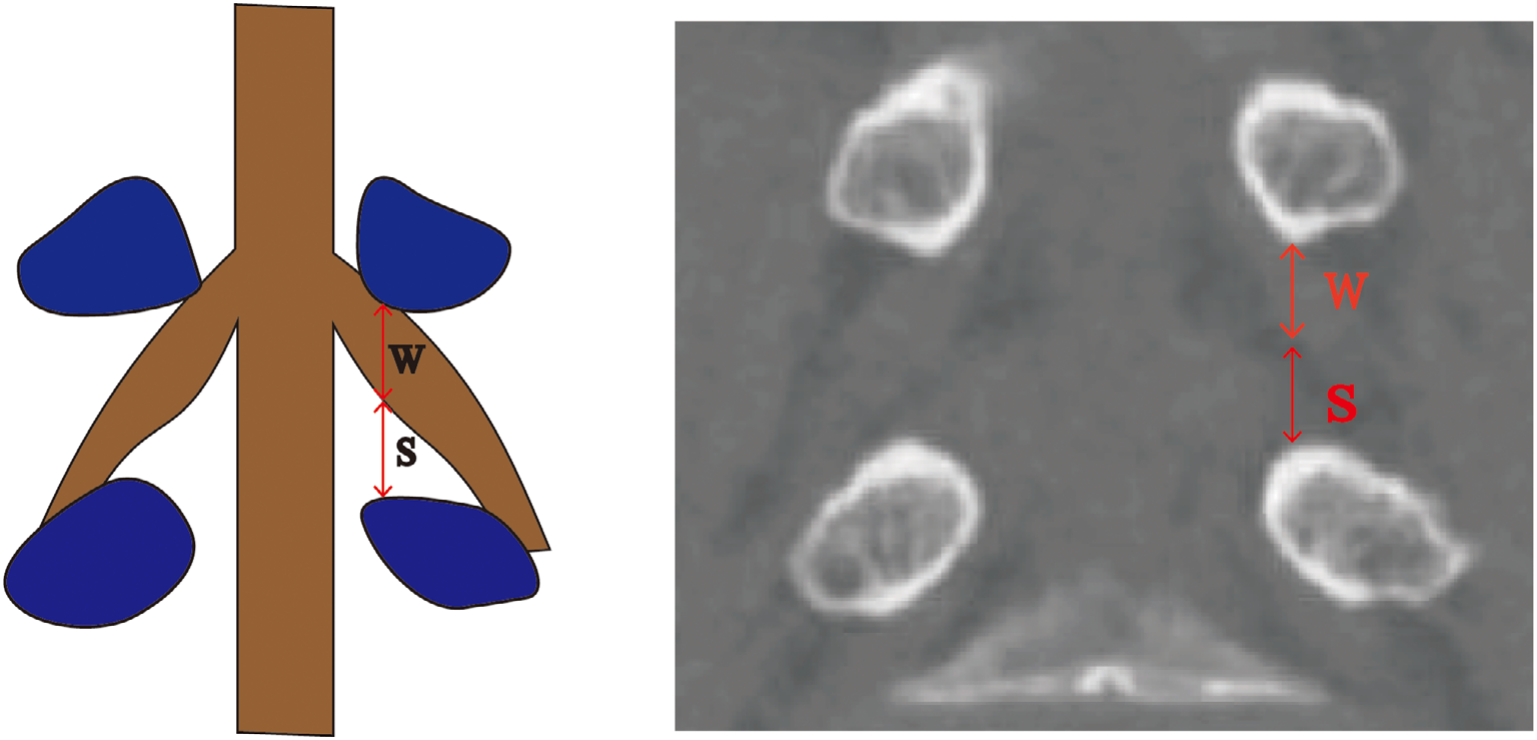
Examples of the parameters measured on the coronal plane of the computed tomography (CT) at the cutting plane of the pedicle. W: Exiting nerve root height; S: Nerve root–pedicle distance.
